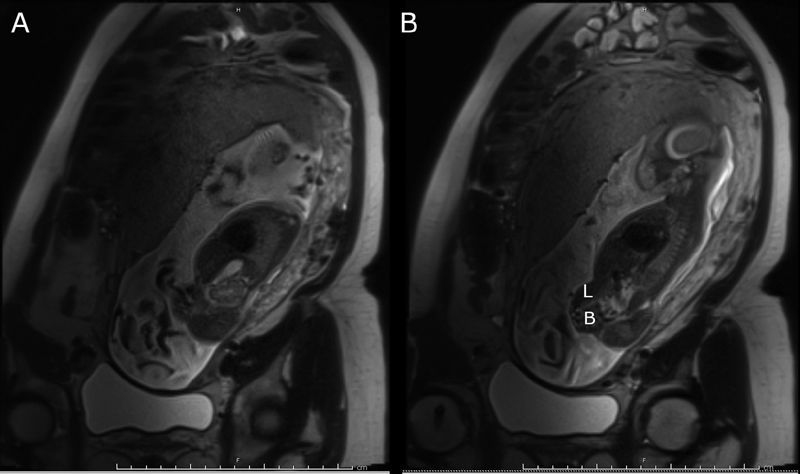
Prenatal MRI demonstrating omphalocele containing liver (L) and bowel (B), absent bladder, and terminal myelocystocele (not pictured on these slices). MRI, magnetic resonance imaging.
